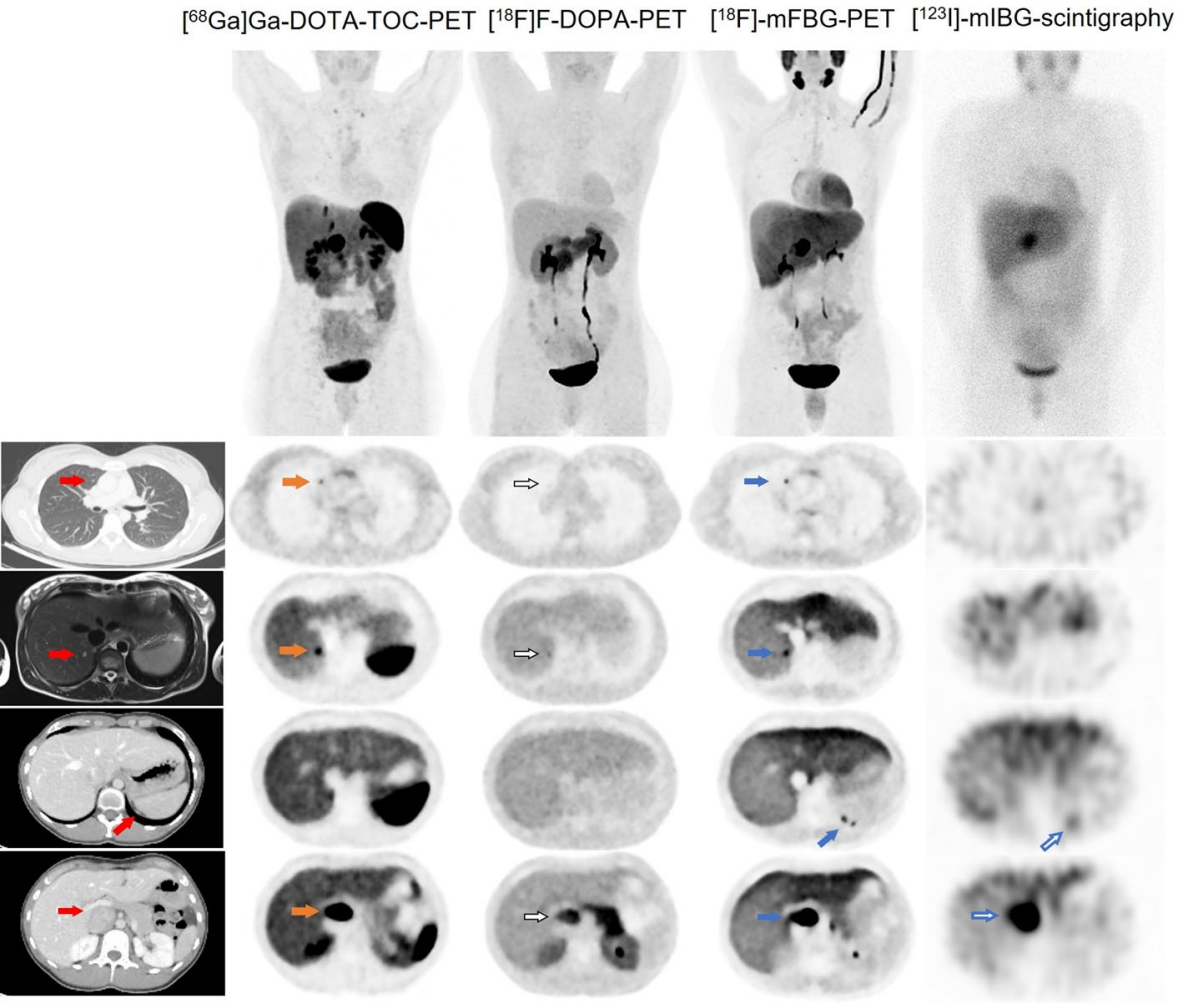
Four molecular imaging modalities and corresponding morphologic imaging in a case with suspected metastatic pheochromocytoma. Columns display different imaging methods: Column 1– morphologic images with CT or MRI (red arrows), Column 2– somatostatinreceptor-PET with [^68^Ga]Ga-DOTA-TOC (orange arrows), Column 3– [^18^F]F-DOPA-PET (open black arrows), Column 4– [^18^F]-mFBG-PET (blue arrows) and Column 5– [^123^I]-mIBG-scintigraphy (open blue arrows). Row 1 shows maximum intensity projections and a whole-body planar image, respectively, and rows 2–5 transversal images in different planes. Row 2 shows a suspected pulmonary metastasis with uptake on [^68^Ga]Ga-DOTA-TOC-PET and [^18^F]-mFBG-PET, relatively lower uptake and hardly detectable on [^18^F]F-DOPA-PET and no uptake on [^123^I]-mIBG-scintigraphy. Row 3 shows a suspected liver metastasis with uptake on [^68^Ga]Ga-DOTA-TOC-PET and [^18^F]-mFBG-PET, relatively lower uptake and hardly detectable on [^18^F]F-DOPA-PET and no uptake on [^123^I]-mIBG-scintigraphy. Row 4 shows two suspected metastases between the spleen and diaphragm with uptake on [^18^F]-mFBG-PET and [^123^I]-mIBG-scintigraphy but no uptake on [^68^Ga]Ga-DOTA-TOC-PET or [^18^F]F-DOPA-PET. Row 5 shows a portocaval suspected malignant lesion with uptake on all imaging modalities
